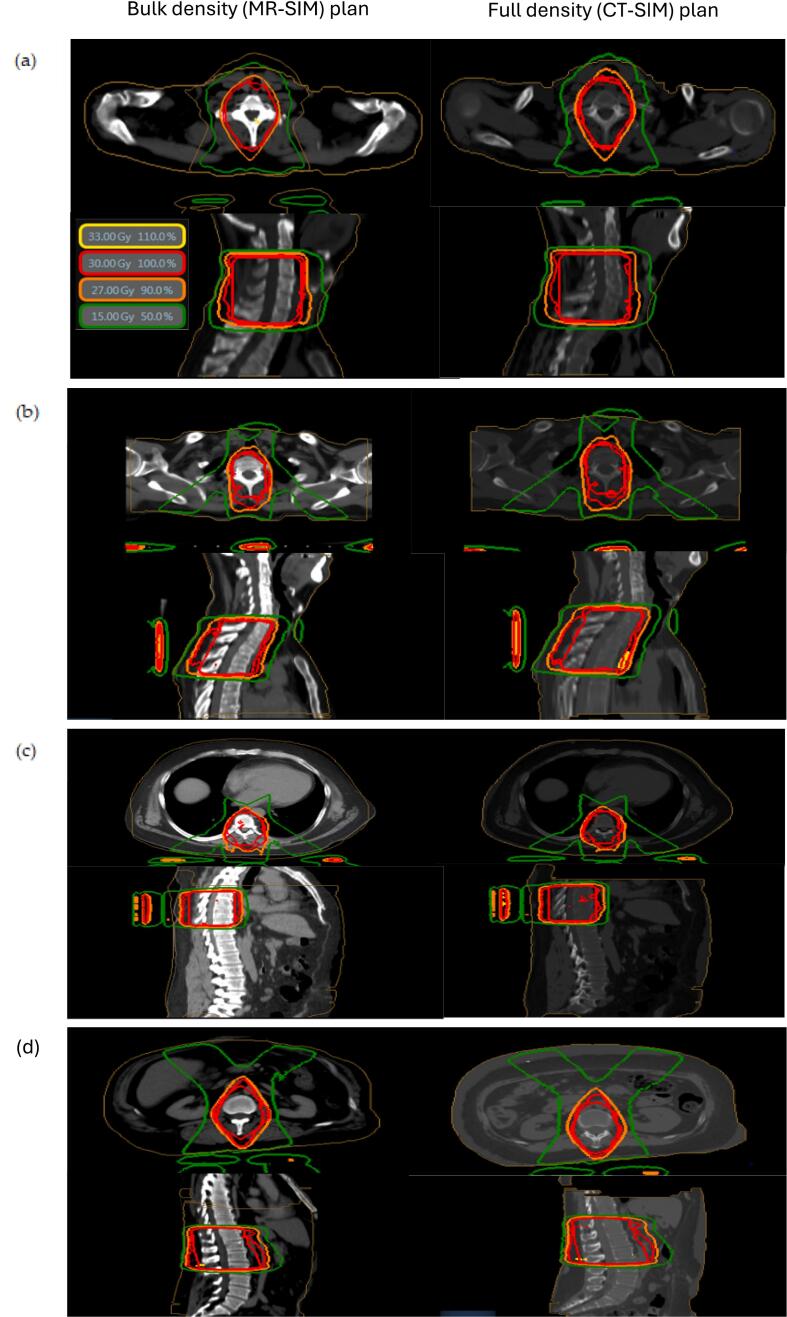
Example dose distributions for (left) bulk density MR-SIM plans and (right) the resulting dose distribution when that plan is transferred to the patient’s full density CT-SIM scan. Plans were generated using the protocols for the (a) cervical spine (C3-C7), (b) superior thoracic spine (T1-T5), (c) mid-thoracic spine (T6-T11), and (d) the inferior thoracic/ lumbar spine (T11-L4) regions.
